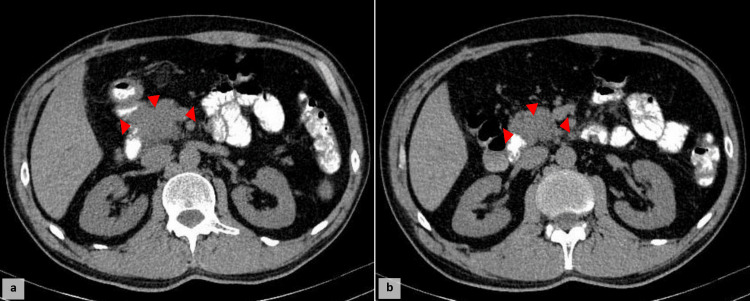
Axial CT scan showing (a) a lesion isodense to the pancreatic parenchyma (arrowhead) and (b) a poorly defined, hypodense lesion in the pancreatic head relative to the adjacent parenchyma during the pancreatic phase (arrowhead).
